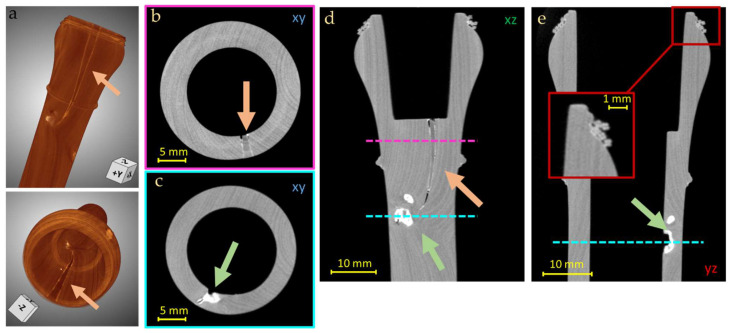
3D render (a) and CT horizontal (b,c), vertical (d) and lateral (e), slices of the Transverse Flute head, where the repaired cracks are visible (orange and green arrows) and a particular of the external cord fixing (e). The colored lines determine the height of the two damages base on the contour of (b,c).
